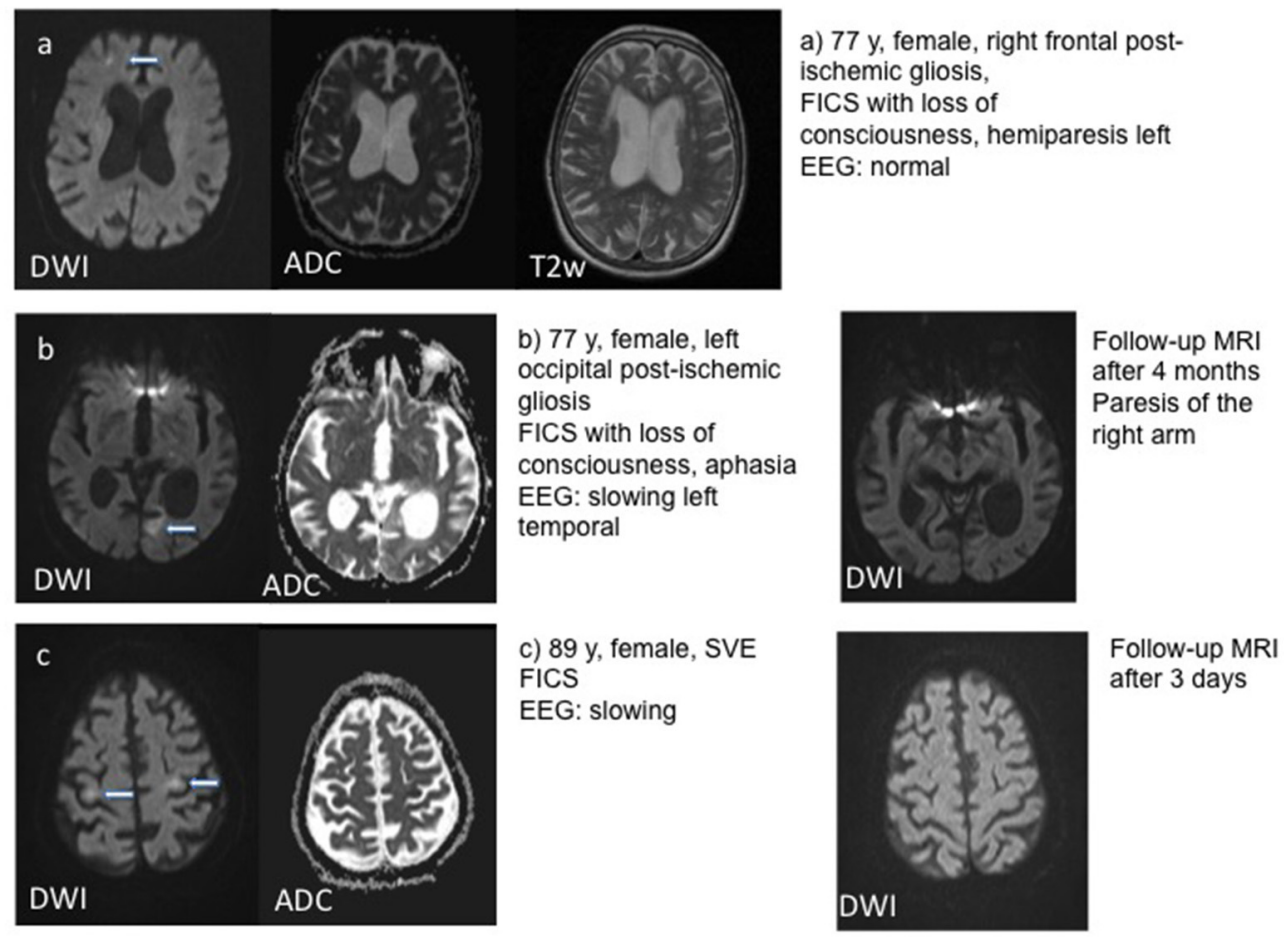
Examples for DWI restrictions in three patients with single focal seizures including follow-up MRI in patients (b) and (c). T2w – T2 weighted imaging.
